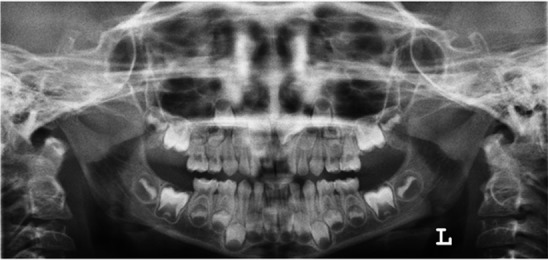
The panoramic radiograph shows no evidence for presence of supernumerary permanent tooth germ in the anterior region. However, rotation of the permanent central teeth germs and development of dens invagination in permanent maxillary right central incisor are suspected.
